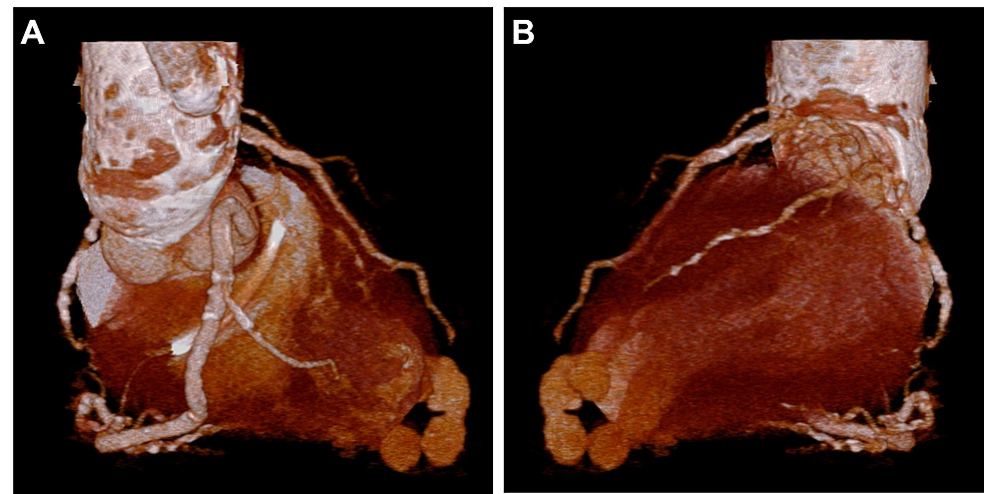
Three-dimensional computed tomography Three-dimensional volume-rendering images clarified the morphology of the pseudoaneurysm. Left anterior oblique 50 (panel A) and 130 (panel B) images show a spiral sequence of pseudoaneurysms starting at the left ventricular apex, resulting in a bead-like appearance.
